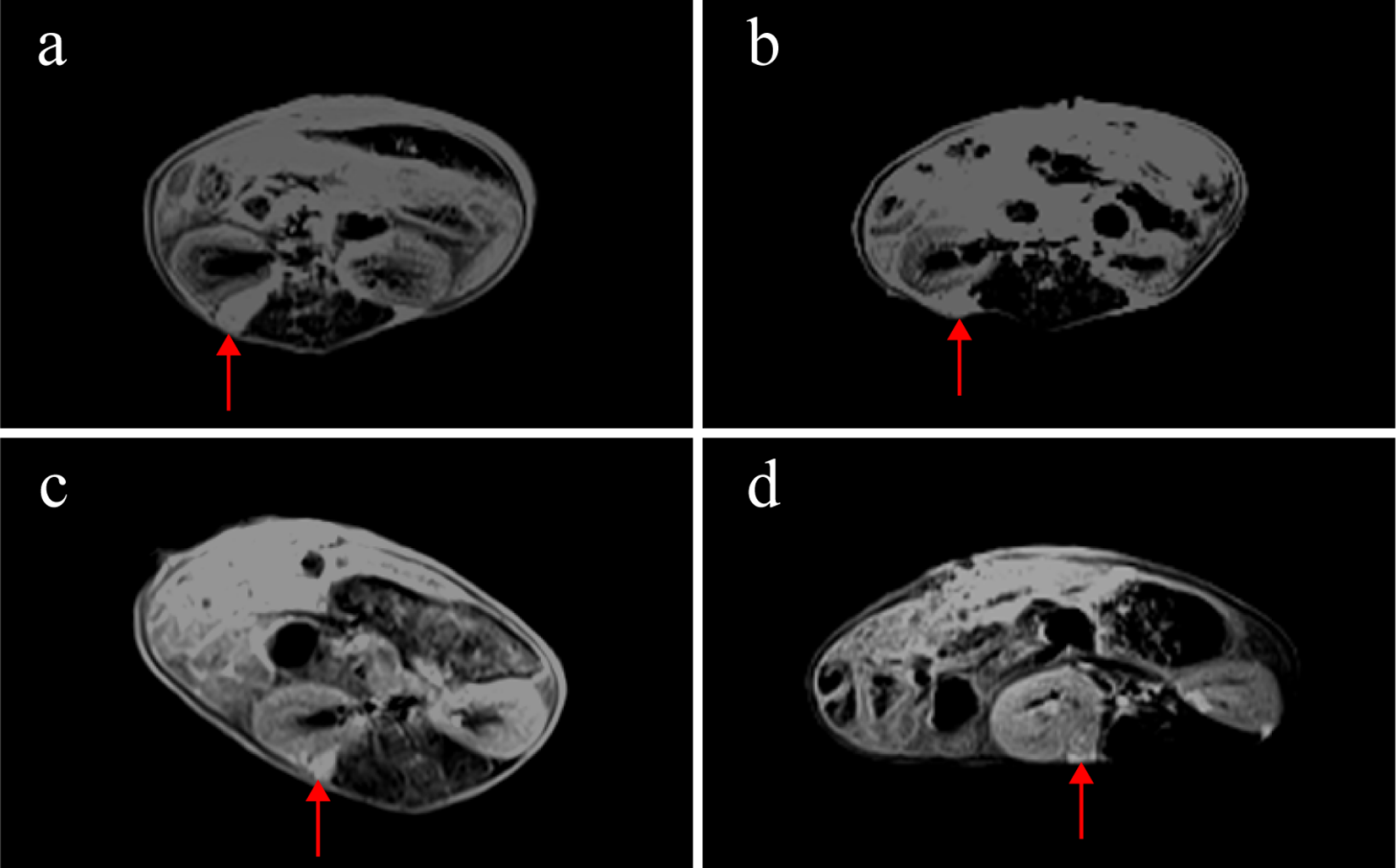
MRI observations of a PDX model in renal capsule (arrow). (a) on the 8th day after establishment, the length of the tumor was 2.5mm, T1WI+C: (b) on the 16th day, the length of the tumor was 2.1mm, T1WI+C; (c) on the 25th day, the length of the tumor was 2.0mm, T1WI+C; (d) on the 32nd day, the length of the tumor was 2.7mm, T1WI+C; T1-weighted contrast-enhanced imaging (T1WI+C).
